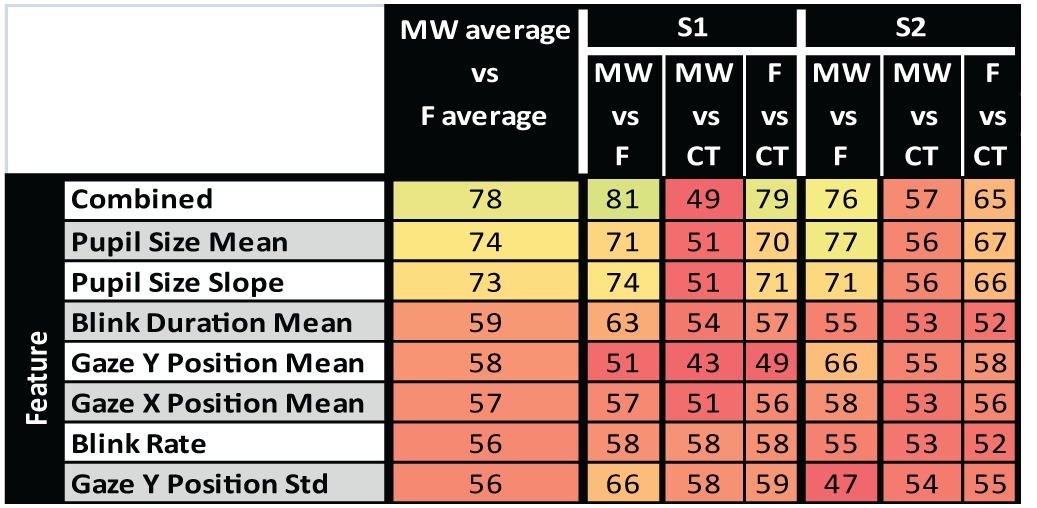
Results of pairwise classification of MW, CT, and F epochs for single oculometric measure and combined oculometric measures.
